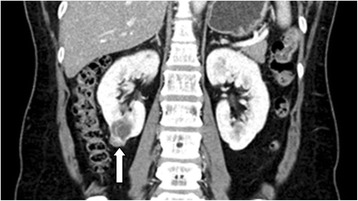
Location of the tumor visualized by computed tomography. The *arrow* shows a well- marginated, multiseptated mass in the lower pole of the right kidney
